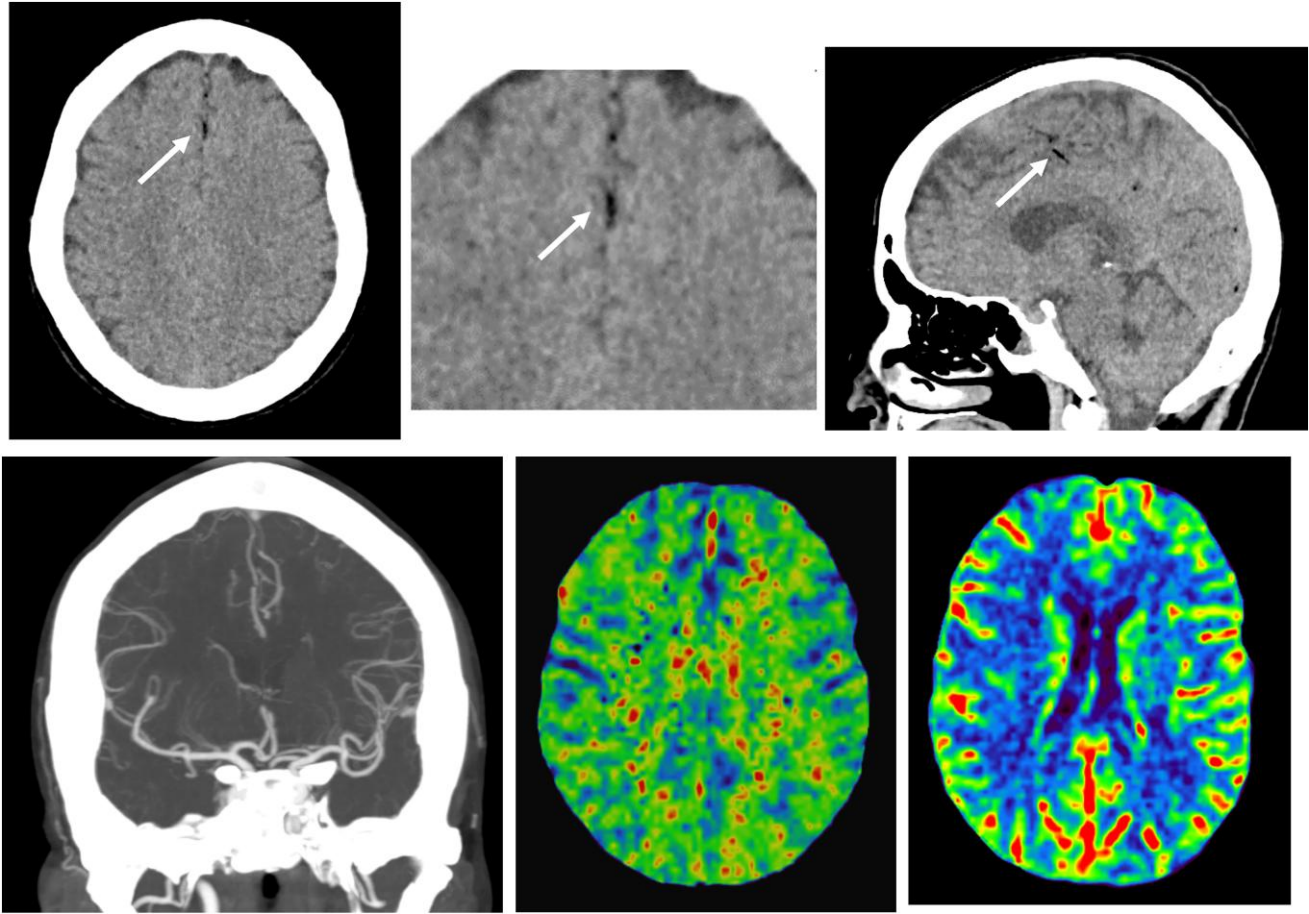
(Above) Head computed tomography. There are no signs of acute ischaemia. Tiny hypodense air bubbles corresponding to pneumocephalus (arrows) are seen in the midline and were omitted in the initial interpretation of the study. (Below) Normal computed tomography brain angiogram and perfusion maps (mean transit time [MTT] and relative cerebral blood volume [rCBV]).
